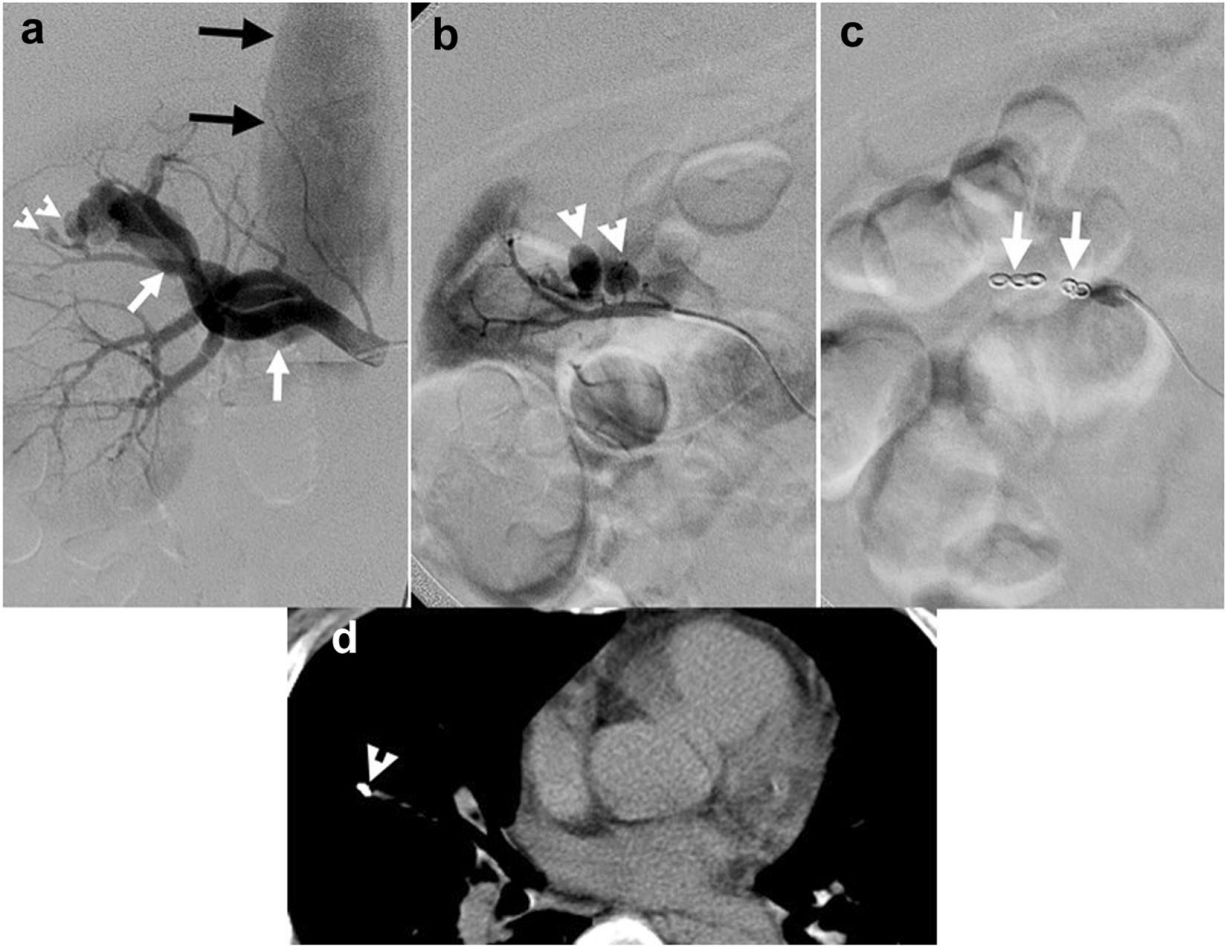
A patient presented with gross hematuria following percutaneous nephrolithotomy due to iatrogenic renal vascular injury. (a) Right renal angiography showing two pseudoaneurysms (arrowheads) and a large arteriovenous fistula (AVF) draining into the right renal vein (white arrows). Note the large size of the inferior vena cava (black arrows) indicating high flow of the AVF. (b) Right renal angiography using superselective catheterization showing pseudoaneurysms (arrowheads). (c) Postembolization angiography for the pseudoaneurysms showing complete cessation of the blood flow distal to the coils (arrows). (d) Computed tomography of the chest showing a coil (arrowhead) at the right lung after a failed attempt of embolization for the high-flow AVF.
